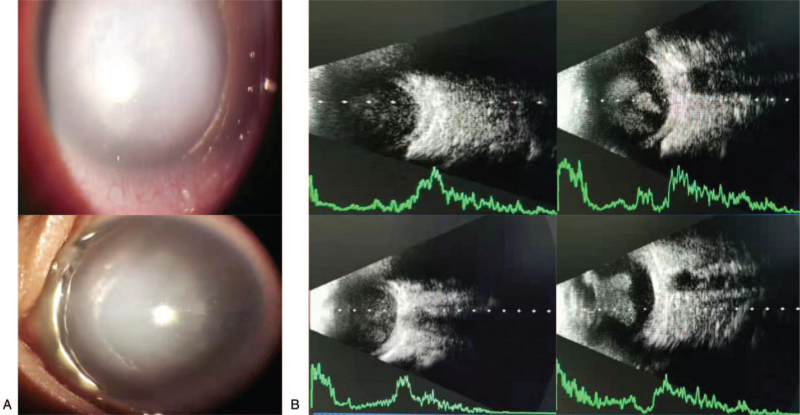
Ocular symptoms in the patient. (A) The result of slit lamp examination. (B) The result of ocular ultrasound.
